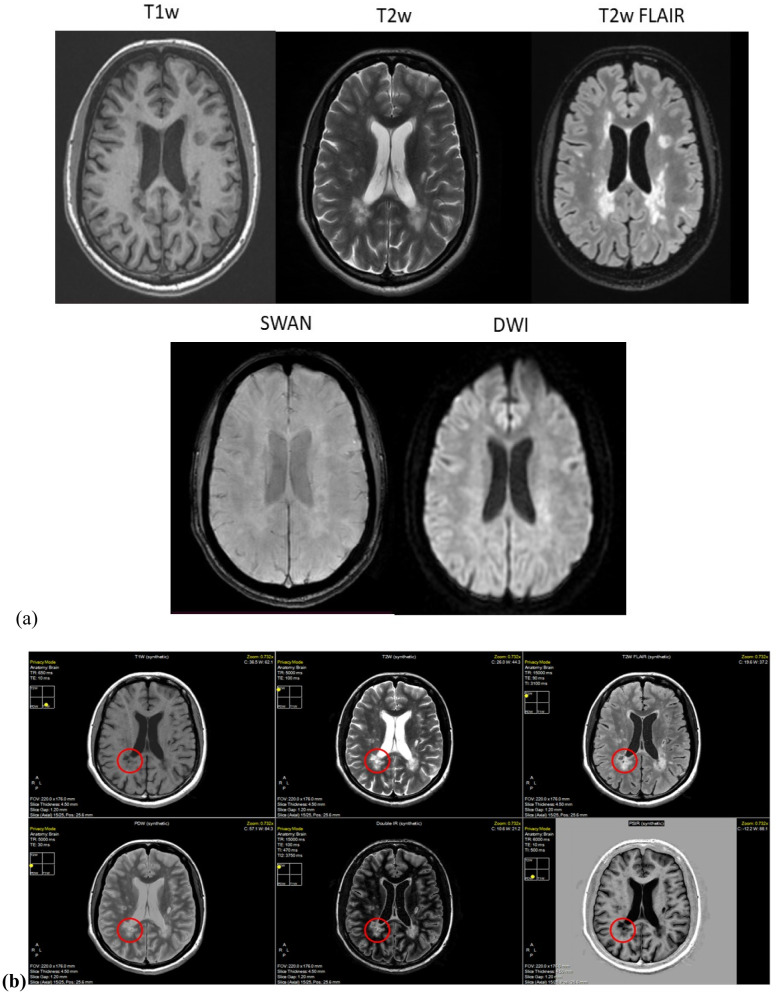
(a) Conventional MRI derived images; (b) Different contrasts generated using the SyMRI software in a 33-year-old female patient with a known case of MS. 69–70 lesions in the supratentorial and seven lesions in the infratentorial regions of the brain were seen on the T2/FLAIR images (min. 3 mm).
